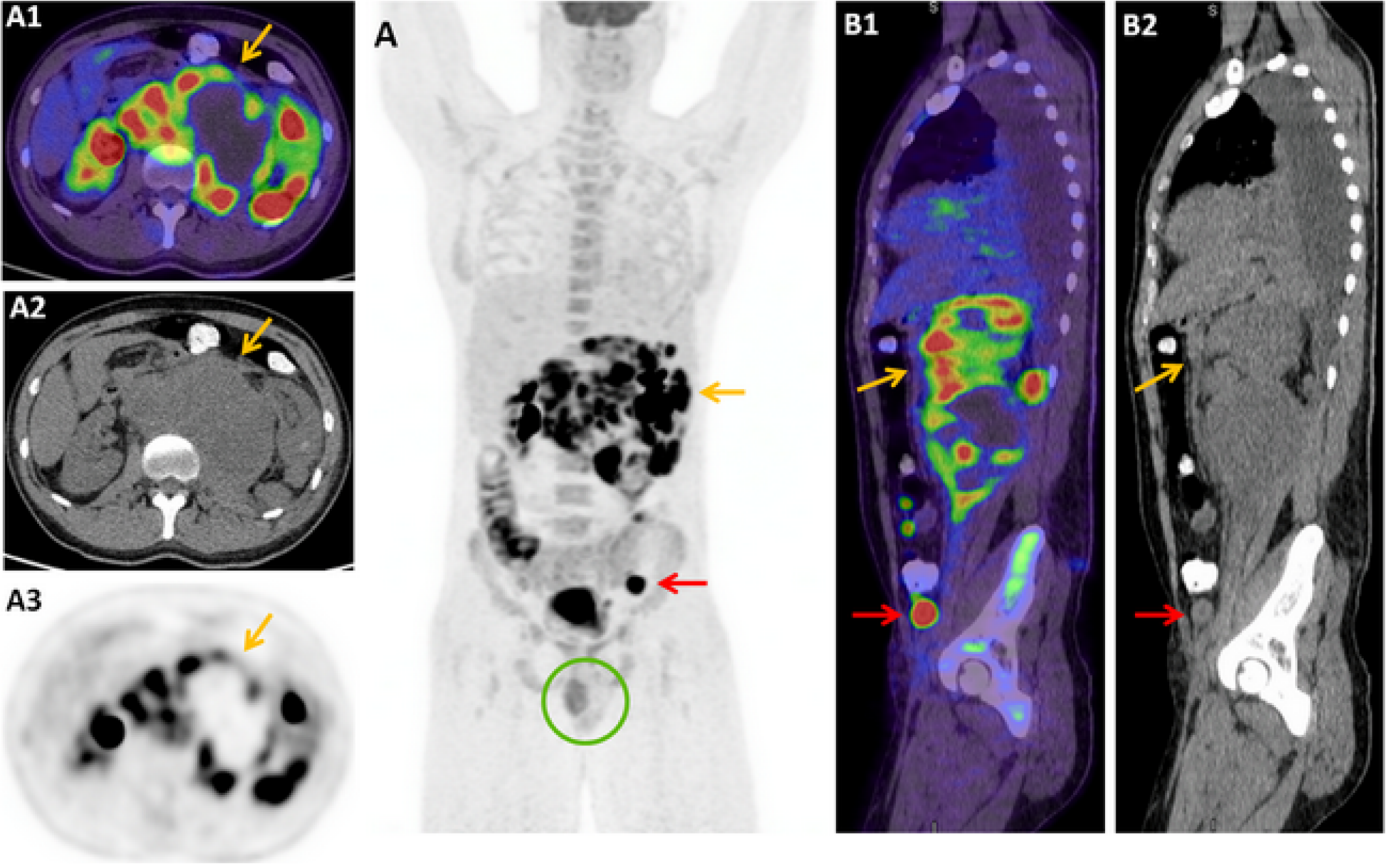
Case One - Staging FDG-PET/CT demonstrates intense FDG-avidity (SUVmax 19) in a bulky and heterogeneous retroperitoneal mass [*orange arrows*] (a1–3, b1–2). A retractile testis [*red arrow*] (b1–2), also intensely FDG-avid (SUVmax 16.4), is shown to be undescended at the time of imaging, situated adjacent to the deep inguinal ring. The maximal intensity projection (MIP) image (a) demonstrates absent left scrotal testicular activity [*circle*]
